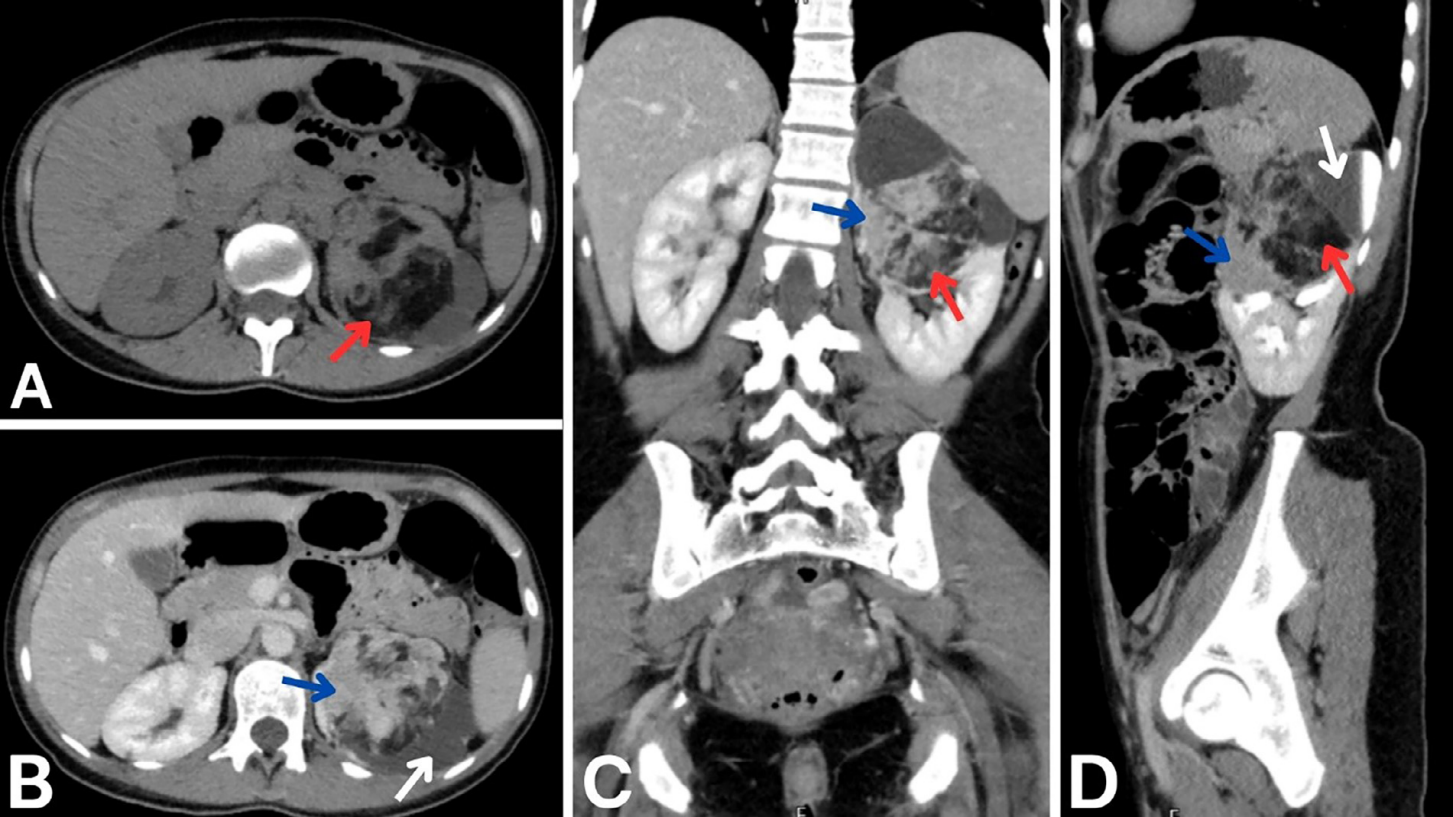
Initial CT imaging with contrast. A: Noncontrast axial plane image showing a large, solid renal mass in the upper and middle poles of the left kidney with mixed densities, including a prominent fat component (red arrow). B: Axial plane in the portal phase showing enhancing soft tissue components (blue arrow) within the mass. Also, it reveals upper calyceal dilation (white arrow) caused by the mass effect from the lesion. C: Coronal plane in the portal phase revealing soft tissue components (blue arrow), and a prominent fat component (red arrow). D: Sagittal plane in the delayed phase highlighting the fat component (red arrow), upper calyceal dilation (white arrow) and soft tissue components (blue arrow).
